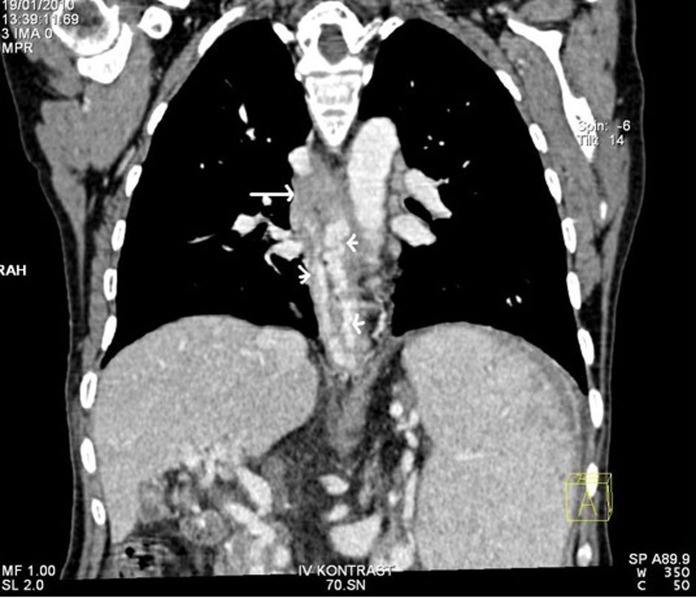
CT of the chest after the intravenous administration of contrast material confirmed the presence of esophageal mass (tall arrow) and varices (short arrows).
